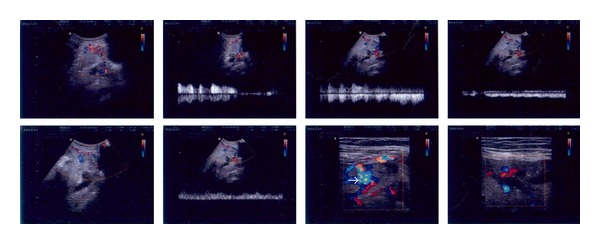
Ultrasound feature of percreta, in 37th week of gestation. Arrow shows lacunas in placenta.
